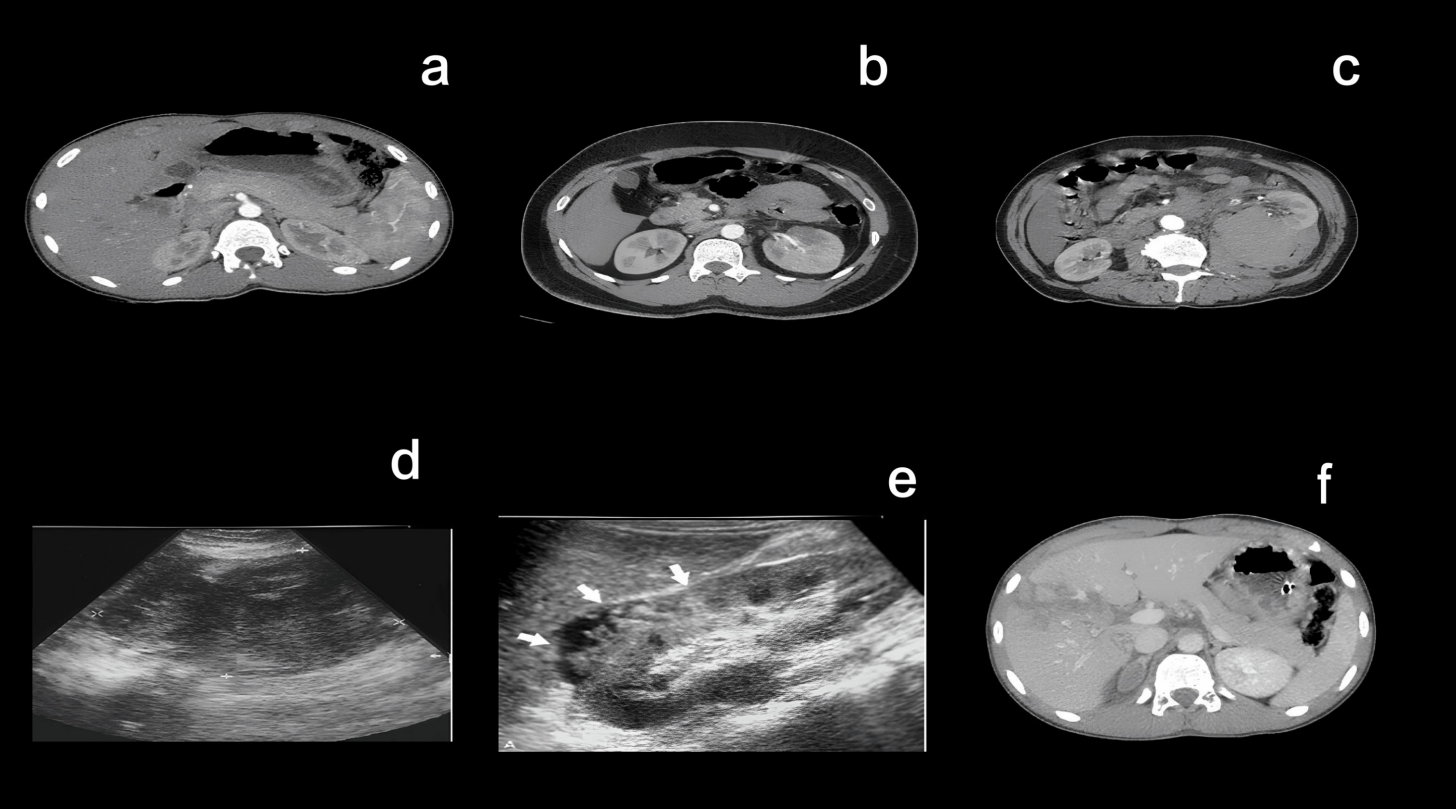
Imaging findings in renal trauma (a) Axial contrast-enhanced CT image showing a Grade I renal injury with a small subcapsular hematoma and intact renal parenchyma. (b) CT image demonstrating a Grade II renal injury characterized by a superficial cortical laceration (<1 cm depth) without urinary extravasation. (c) CT image showing a Grade III renal injury with a deeper laceration (>1 cm) extending into the medulla but without collecting system involvement. (d) USG image revealing a well-defined hypoechoic subcapsular collection, consistent with a small perinephric hematoma. (e) USG image displaying multiple hypoechoic and irregular areas (arrows) corresponding to parenchymal disruption and early liquefaction, suggestive of a higher-grade renal injury. (f) Contrast-enhanced CT image depicting a Grade IV renal injury with a deep laceration extending into the collecting system and perinephric fluid collection.
